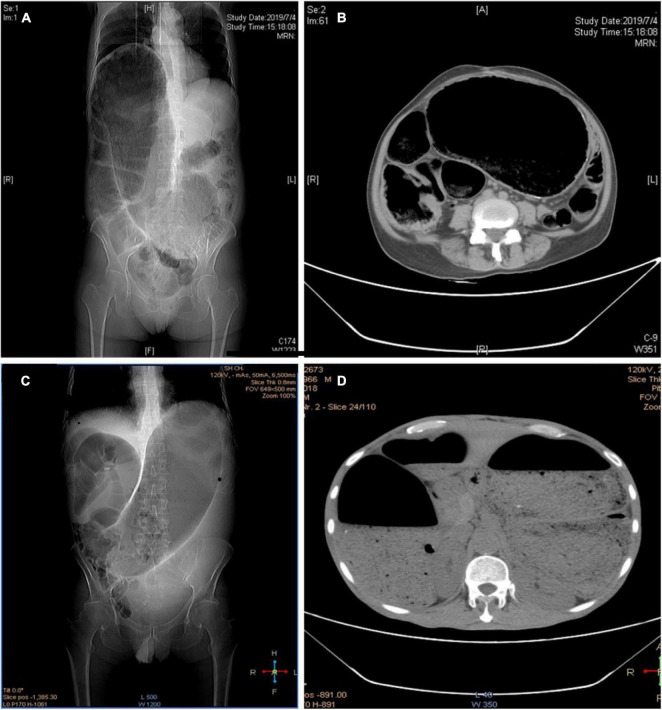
(A,B) CT image of iatrogenic megacolon caused by colonic anastomotic stenosis; (C,D) CT images of iatrogenic megacolon after radiotherapy for rectal cancer.
